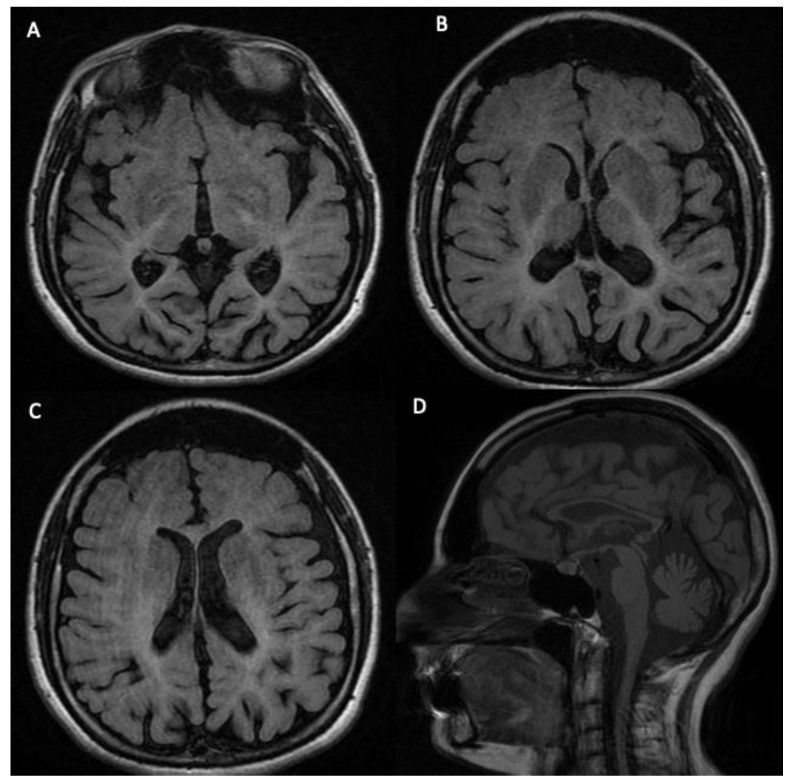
The brain MRI sequences show diffuse, bilateral, and relatively symmetrical cerebral white-matter hyperintensities, consistent with areas of hypomyelination, particularly evident in the subcortical and periventricular areas in T2-weighted and FLAIR images (A–C). In the same sequences, the extra-axial cerebrospinal fluid (CSF) spaces (including sulci, fissures, and ventricles), especially along the high-convexity area, are dilated. Sagittal T1-weighted image (D) shows a marked thinning of the corpus callosum, a verticalization of the tentorium, and olives and pontocerebellar hypoplasia. No signal/morphology alterations are evident in the cervical medulla.
